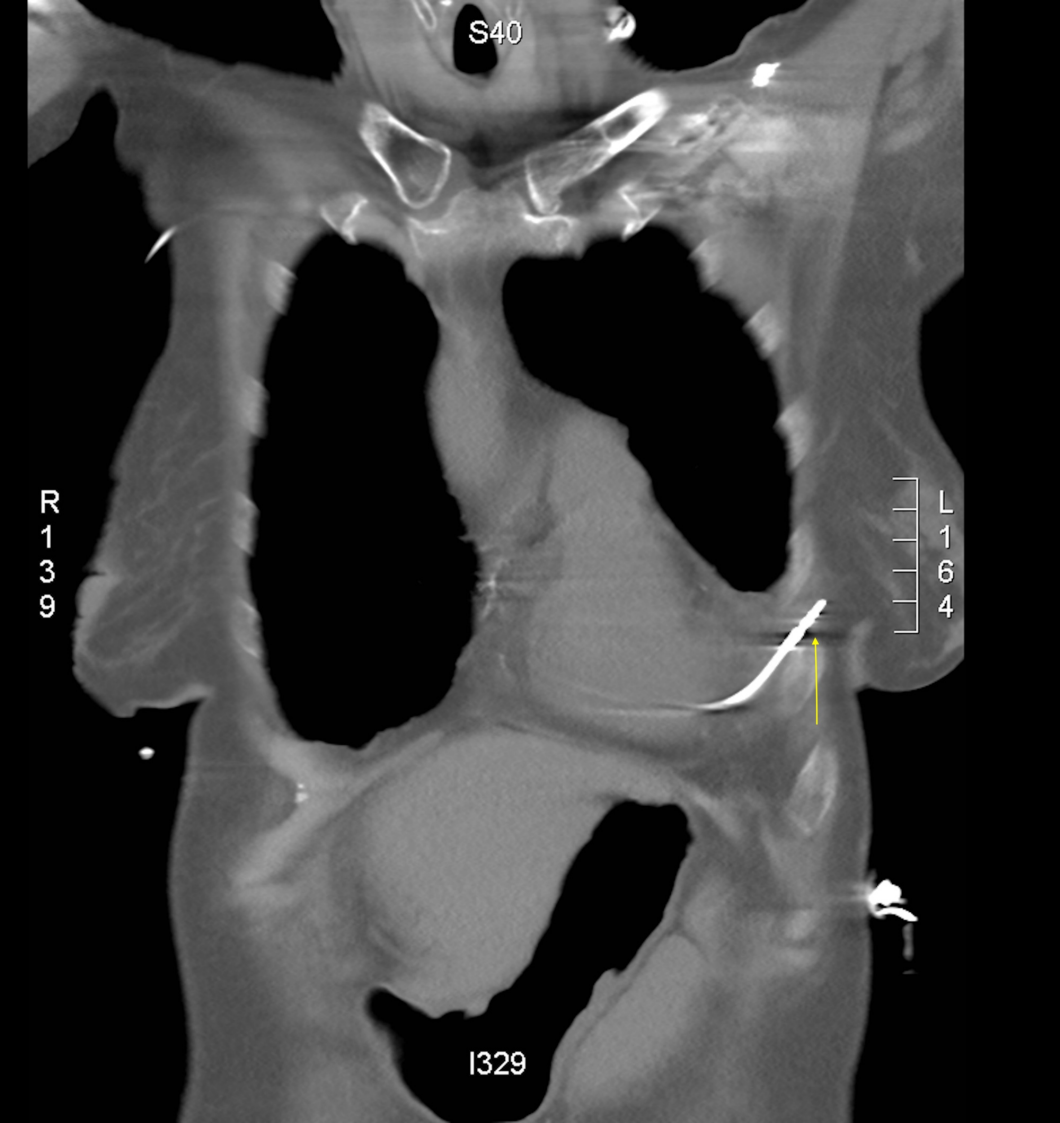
CT chest without contrast This image is a sagittal view of a chest CT showing the pacemaker lead penetrating through the myometrium, pericardium, intercoastal muscle and into the chest well.
